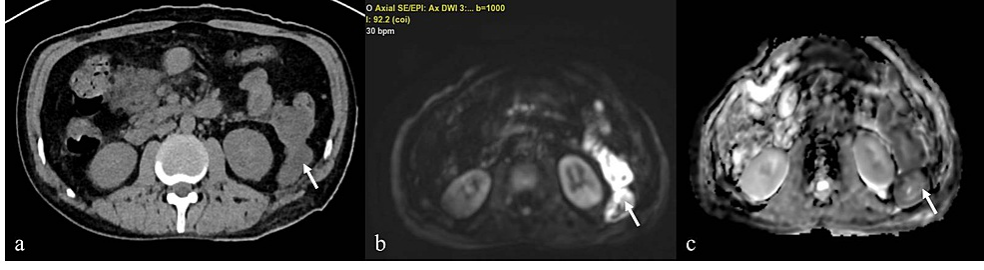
A 33-year-old-male presented with alcohol-induced acute pancreatitis. (a) Axial non-contrast CT shows heterogeneous collection along the left paracolic gutter without air (arrow). (b, c) Diffusion-weighted MR images and corresponding ADC map showing diffusion restriction in the collection (arrow). He had infected collection confirmed by fine-needle aspiration.
